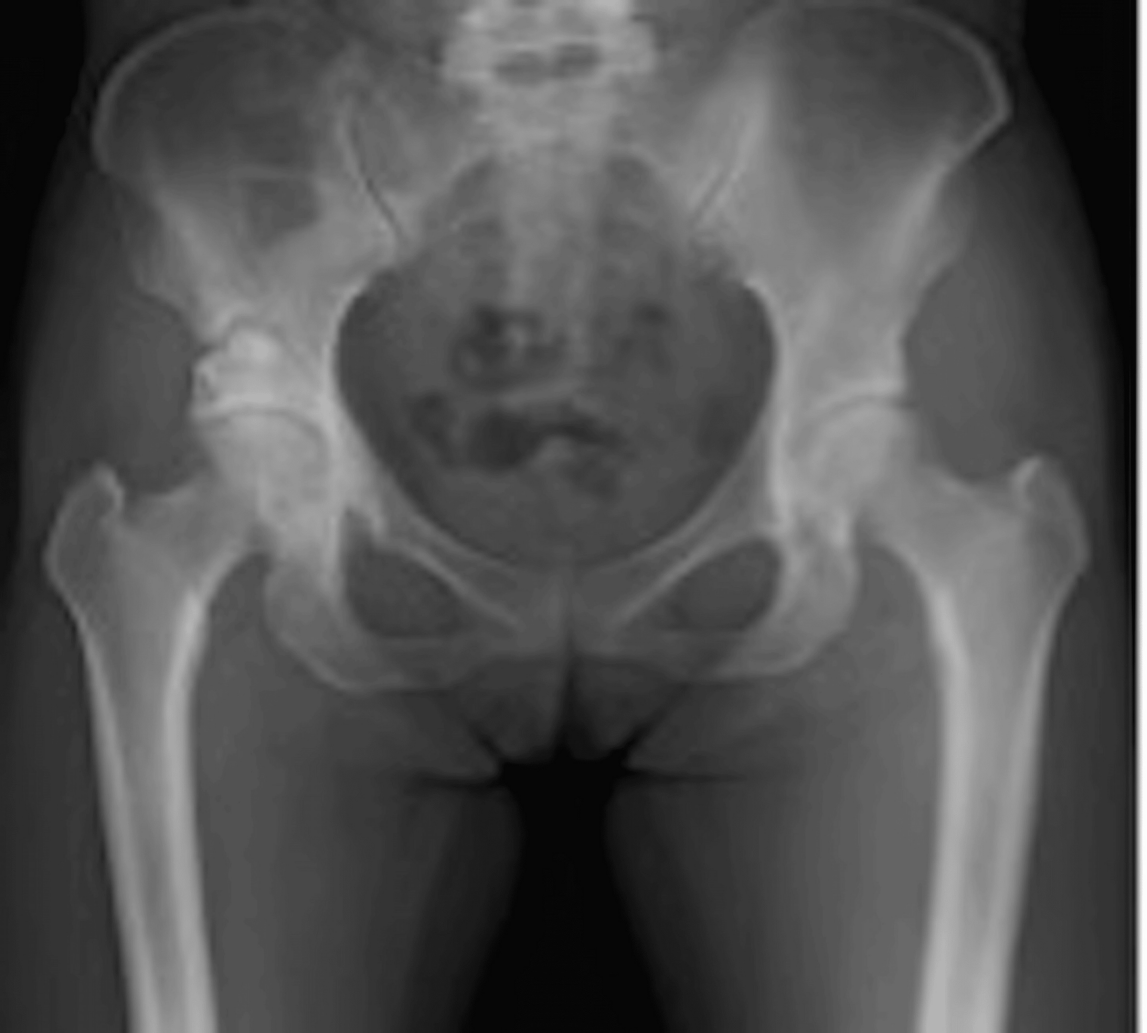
Radiographs at six months postoperatively of case 1. Complete bone union was observed at the osteotomy site. CE angle: 30.4°, ARO: 5.5°, Sharp's angle: 50.3°, AHI: 95.4%. CE, center–edge; ARO, acetabular roof obliquity; AHI, acetabular head index
